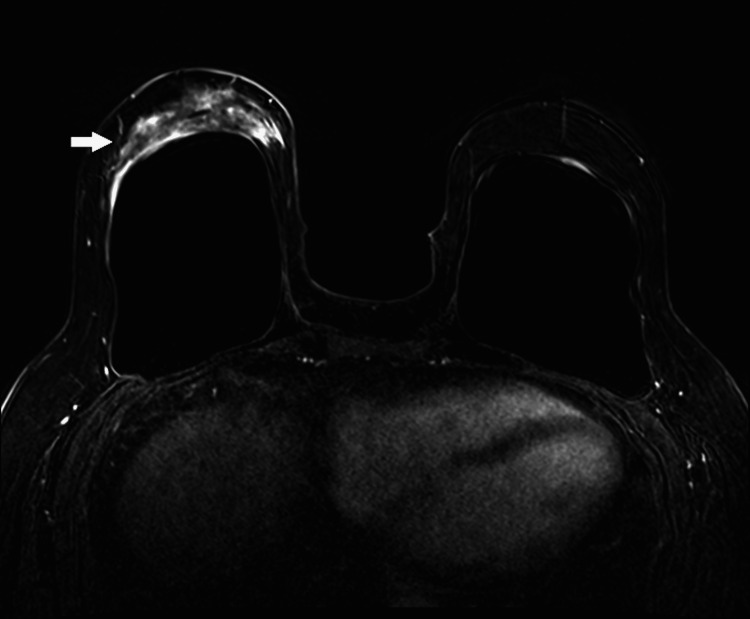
Post-contrast axial subtraction MRI image demonstrating heterogeneous regional non-mass enhancement in the inferior right breast (arrow), markedly asymmetric to the contralateral breast, which corresponded with the site of asymmetry on mammogram.
